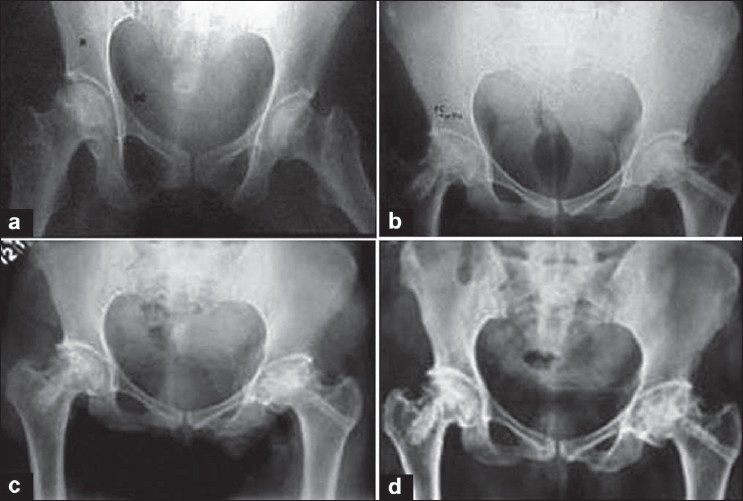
(a) Preoperative X-rays of the pelvis with both hips of a young lady shows osteonecrosis of both femoral heads. (b) Postoperative X-rays of the same patient at 2 years, where vascular pedicle graft from deep circumflex iliac artery (right side) and fibular bone graft (left side) was done. (c) Postoperative X-ray of the same patient at 5 years showing good vascularization of the right femoral head and postoperative. (d) X-ray at 10 years showing good revascularization of the femoral heads without any collapse or arthritic changes.
